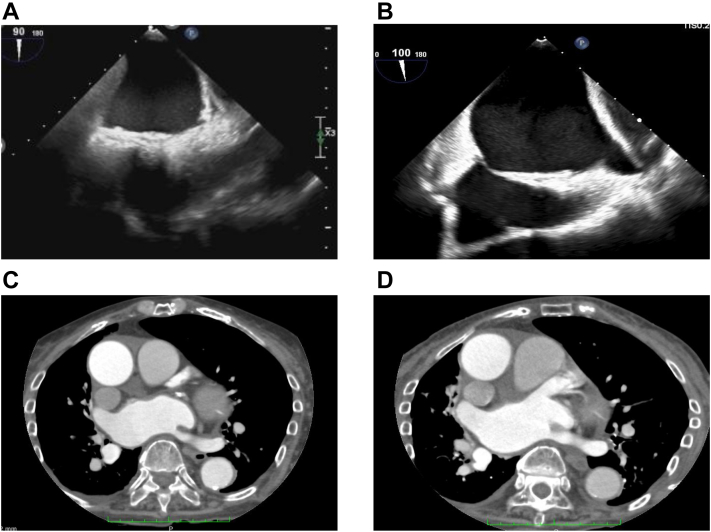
Comparison of Interatrial Septum and Left Atrial Enlargement Between the Prior and Current Procedures (A) TEE in the bicaval view immediately after septal device implantation, showing the interatrial septum almost entirely occupied by the closure devices. (B) TEE in the bicaval view at the time of transcatheter edge-to-edge repair, demonstrating stretching of the interatrial septum. (C) CT imaging obtained at the time of septal closure, showing normal left atrial dimensions. (D) CT imaging 1 year later, demonstrating marked left atrial enlargement and stretching of the interatrial septum. CT = computed tomography; TEE = transesophageal echocardiography.
